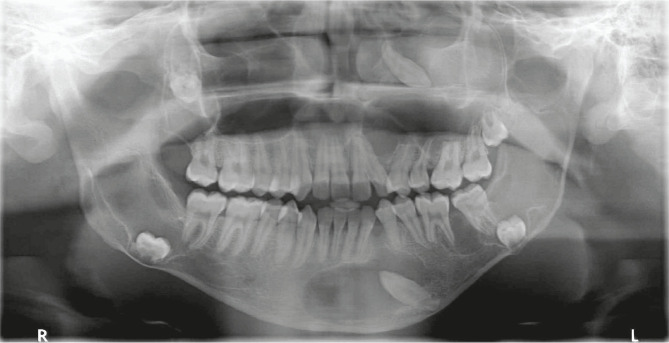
Panoramic radiograph showing multiple cyst-like lesions associated with unerupted teeth in the jaws.
